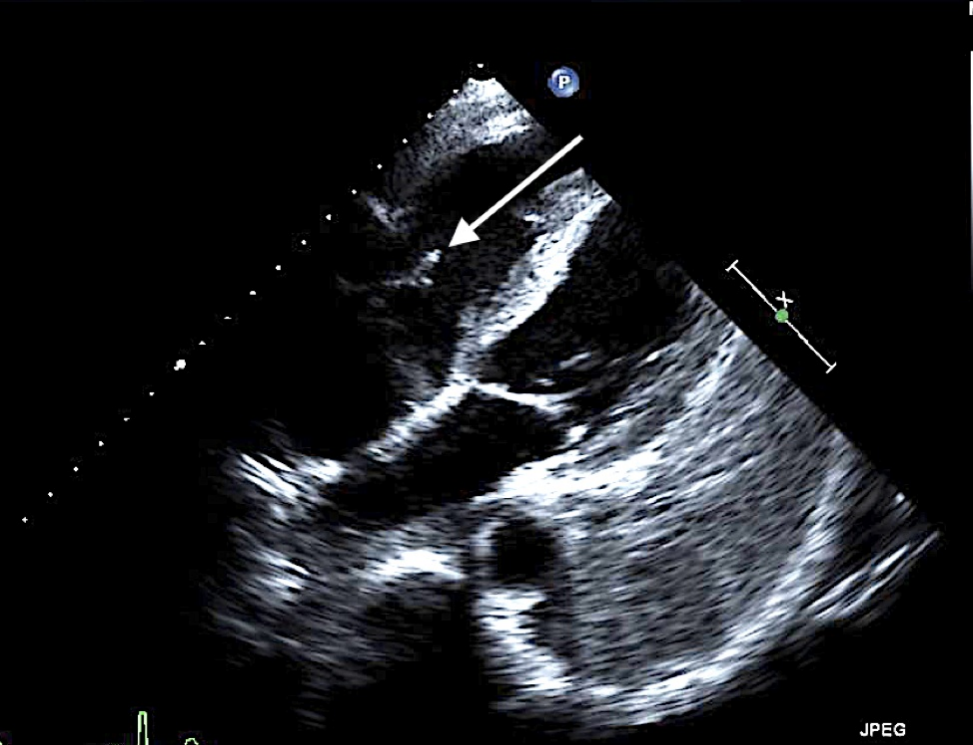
Transthoracic echo cardiogram showing mobile echo density noted on the anterior tricuspid leaflet measuring 0.9 x 0.3 cm (white arrow)
